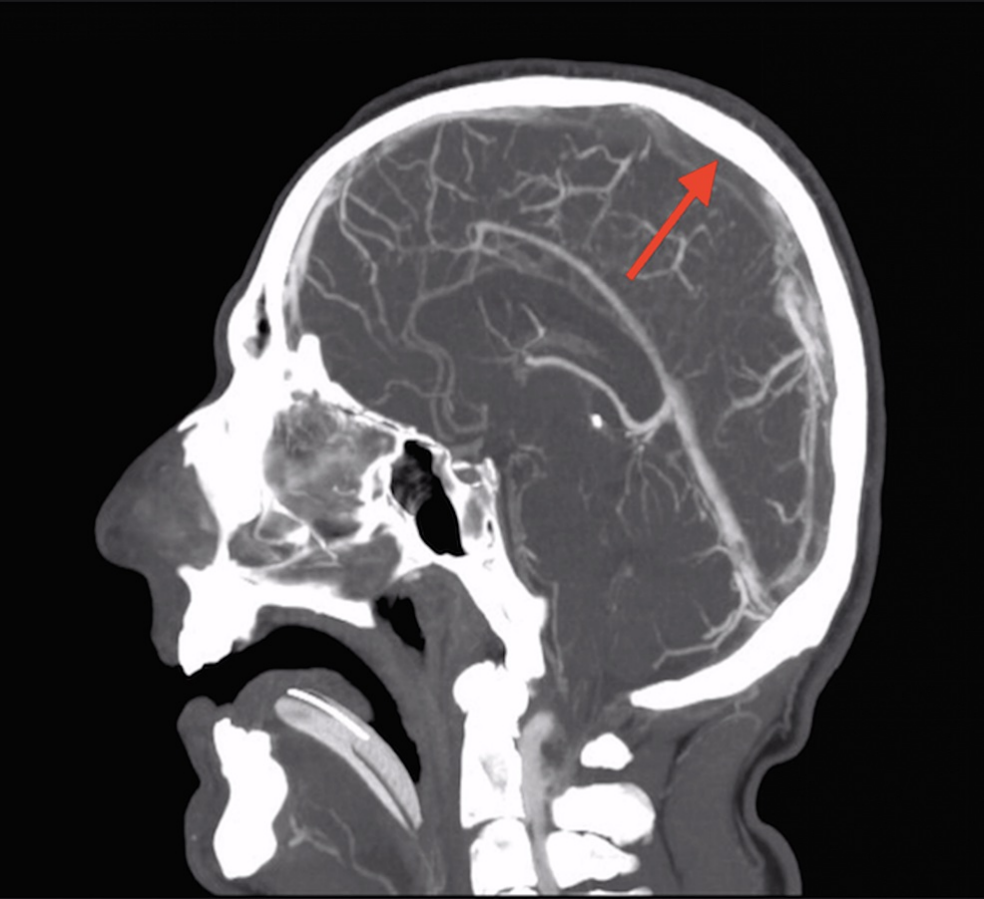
CT venogram of the brain (sagittal section). The red arrow is pointing toward the superior sagittal sinus where there is a massive thrombus (no contrast filling)
